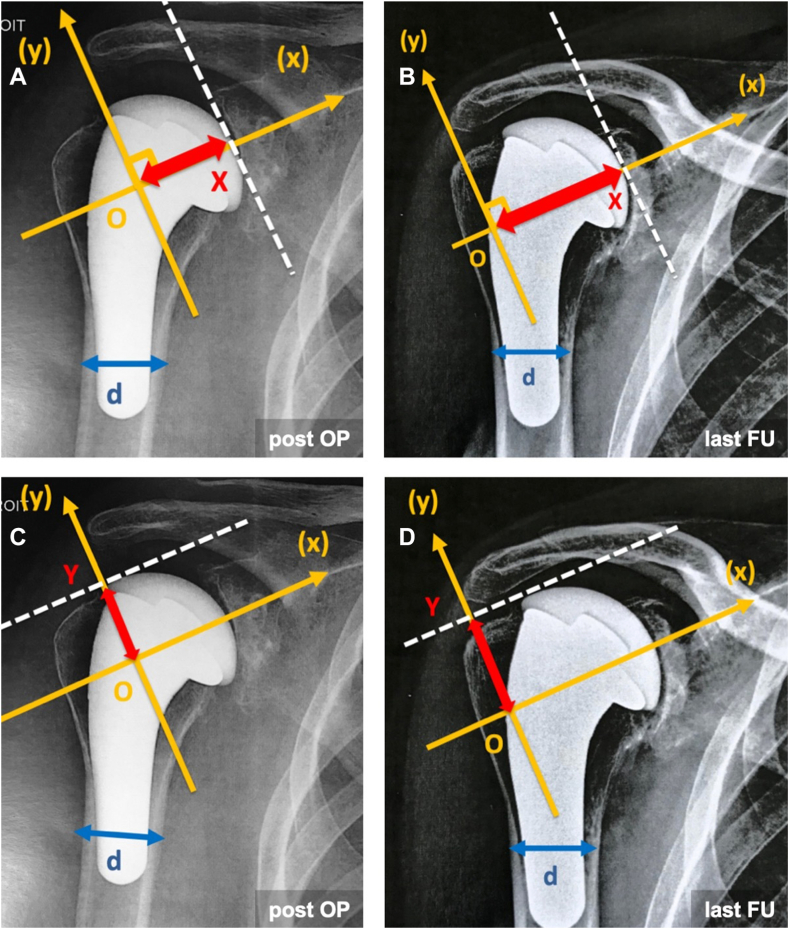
Measurements of humeral medialization and humeral upward migration - (x) is the axis of the supraspinatus fossa. (y) is the orthogonal line to (x) tangent to the lateral border of the acromion. Point O: intersection of (x) and (y). Point X: intersection of (x) and the orthogonal line to (x) tangent to the medial border of the prosthetic humeral head. Point Y: intersection of (y) and the orthogonal line to (y) tangent to the upper part of the humeral head. d: humeral shaft diameter from lateral to medial cortex at the inflexion point of the calcar. To overcome the problem of scaling, OX and OY measurements have been normalized to d. Medialization: ΔOX/d = OX(last FU)/d(last FU) – OX(post-op)/d(post-op). Upward migration: ΔOY/d = OY(last FU)/d(last FU) – OY(post-op)/d(post-op). (A) Measurement OX (post-op) on the immediate post-operative AP X-rays. (B) Measurement OX (last FU) on the last FU AP X-rays. (C) Measurement OY (post-op) on the immediate post-operative AP X-rays. (D) Measurement OY (last FU) on the last FU AP X-rays. *AP*, anteroposterior; *FU,* follow-up.
